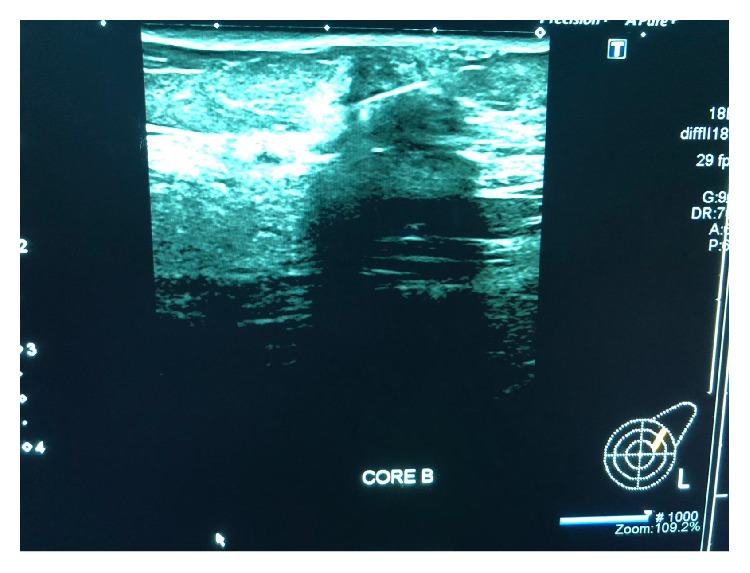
Ultrasound-guided core biopsy of the second lesion (14-gauge needle).
